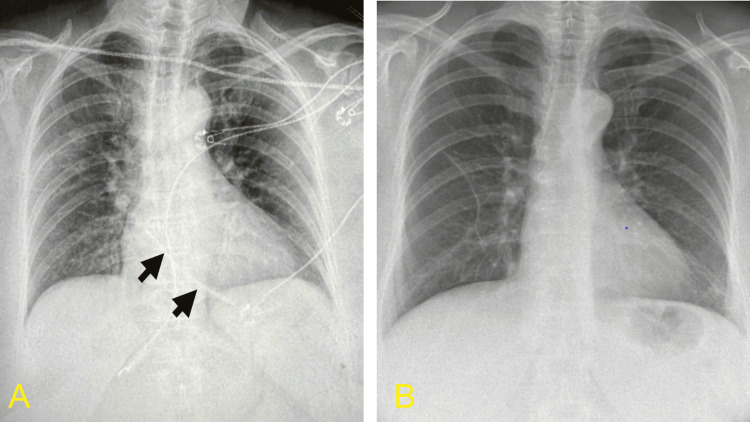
(A) CXR demonstrating the migrated and fragmented catheter segment lodged within the right atrium (black arrowheads). (B) Post-retrieval chest radiograph confirming complete removal of the intracardiac foreign body. CXR, chest X-ray.
